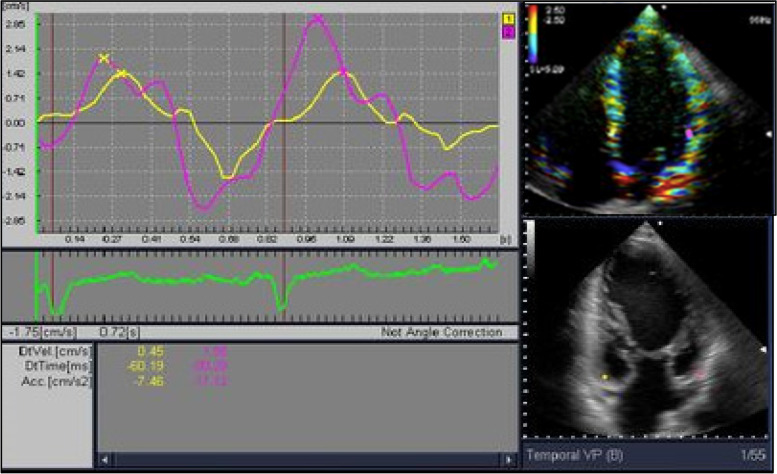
Strain measurement. Tissue Doppler echocardiography, strain rate, and strain profiles of a normal subject obtained from an apical four chamber view. Time is on the X-axis. The waveform consists of a first positive wave (pre-ejection period), followed by the isovolumic relaxation waves. Longitudinal strain rate profile is obtained from the same region of interest during the same cardiac cycle
